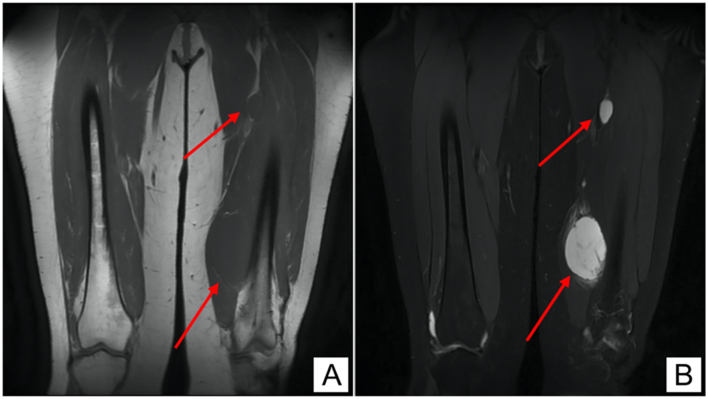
(A) Coronal MRI section showing low signal intensity on T1W imaging in two soft tissue lesions. (B) Coronal MRI section showing high signal intensity on T2W imaging in two soft tissue lesions.
